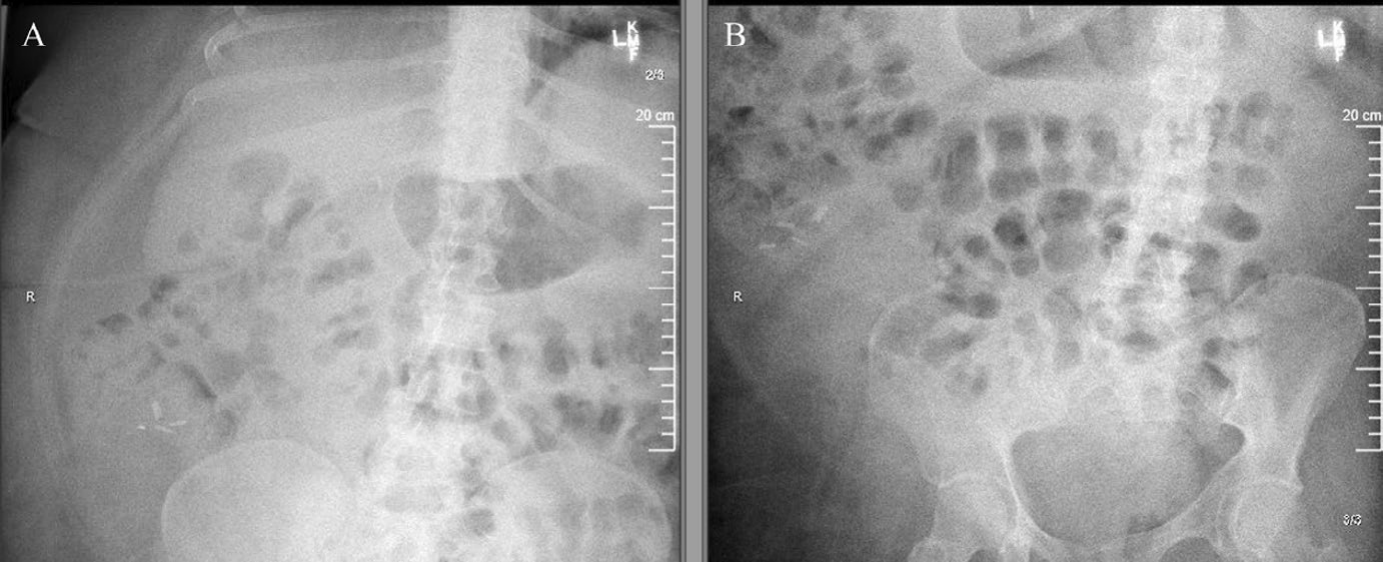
Abdominal radiographs obtained after retrieval. (A) The left image demonstrates post‐colonoscopic retrieval, confirming the successful removal of the foreign body. (B) The right image further highlights the successful retrieval, with evidence of normal bowel gas patterns and no signs of obstruction or perforation.
